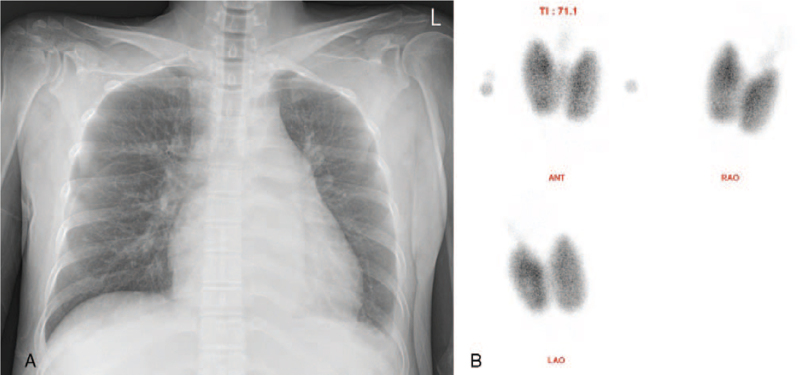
A, A chest radiograph showing mild cardiomegaly and blunted costophrenic angle. B, Technetium-99m thyroid scan demonstrating diffuse bilateral enlargement of thyroid gland with intense homogeneous radiotracer uptake suggesting Graves’ disease.
